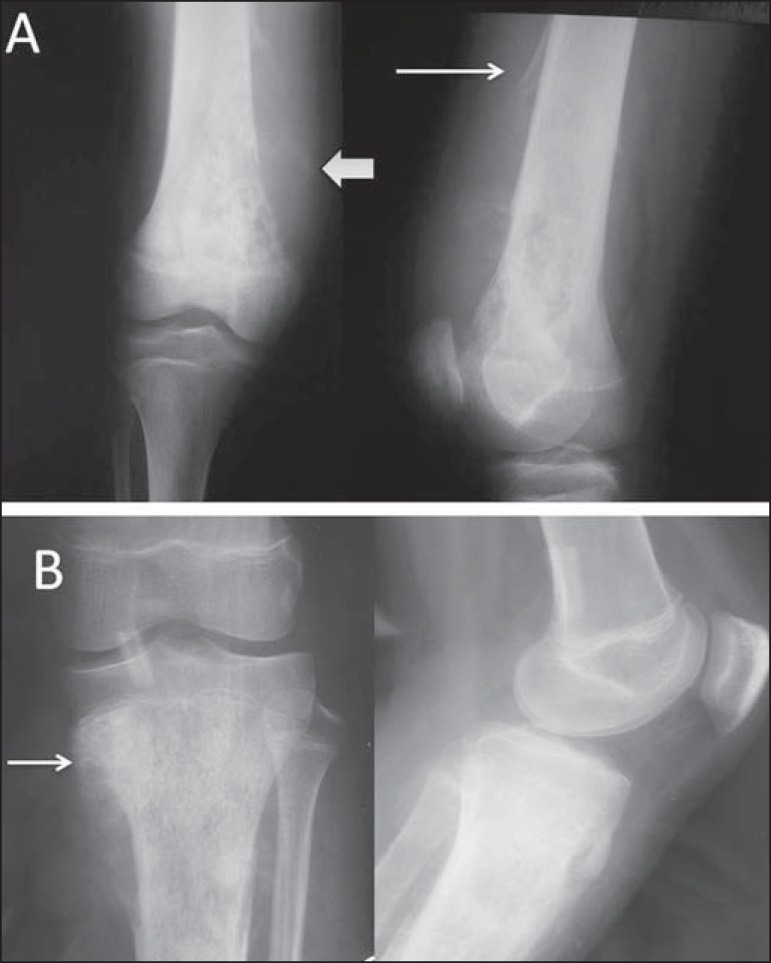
A: Anteroposterior and lateral conventional radiography
